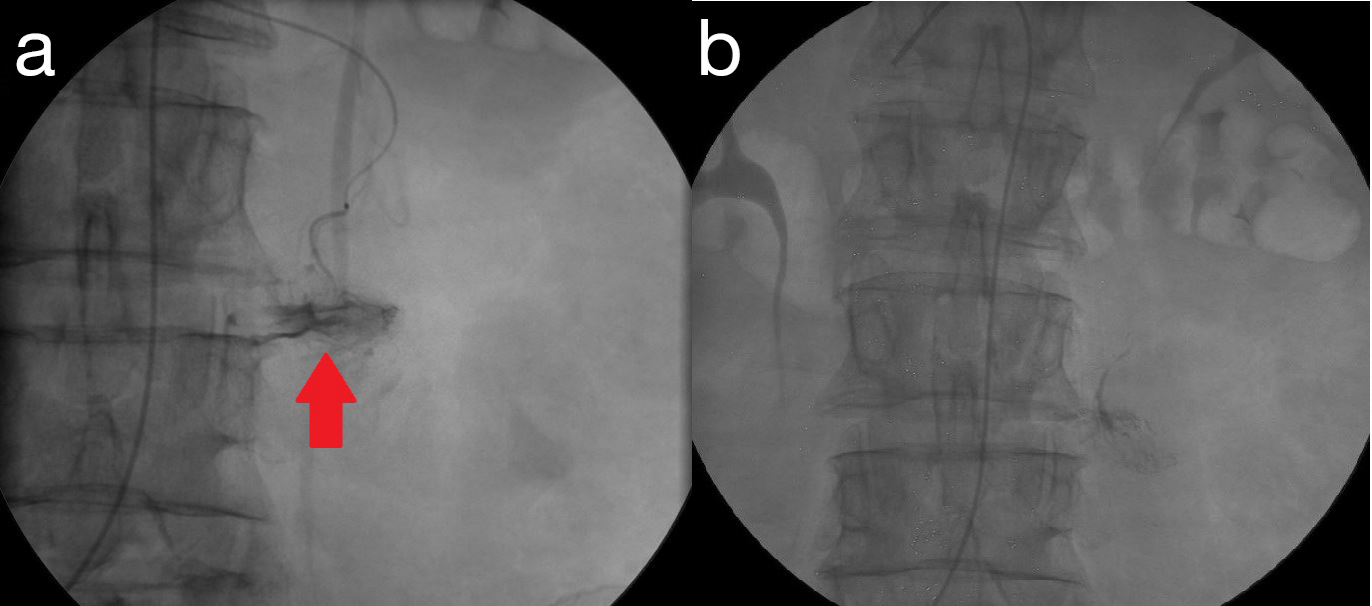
a 73 years old female presenting with melena and anaemia. CT-angiography of the abdomen does not show any sign of active or recent bleeding. DSA demonstrates the presence of an active bleeding from jejunal branches of the superior mesenteric artery (A; red arrow) and successful TAE with 2 ml Glubran 40% is performed (B; the radiopacity within the feeding artery represents Glubran-Lipiodol mixture [the second being iodine-rich], which has stopped blood flow into the “vascular tufts” [no longer opacified]).
